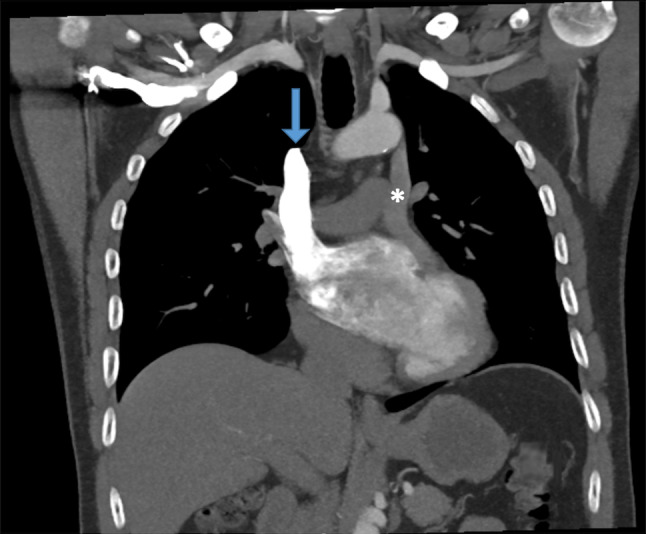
Computed tomography angiography, coronal plane. *Arrow* indicates right superior vena cava (*SVC*) with contrast, draining into the left atrium. *Asterisk* indicates persistent left SVC, draining into a dilated coronary sinus (which is also shown in Fig. 1a of the Question)
